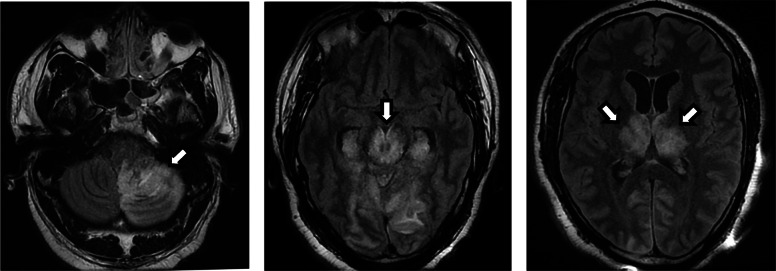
MRI brain without contrast shows severe ischemic injury of cerebellum, brainstem, and thalami (arrows).
